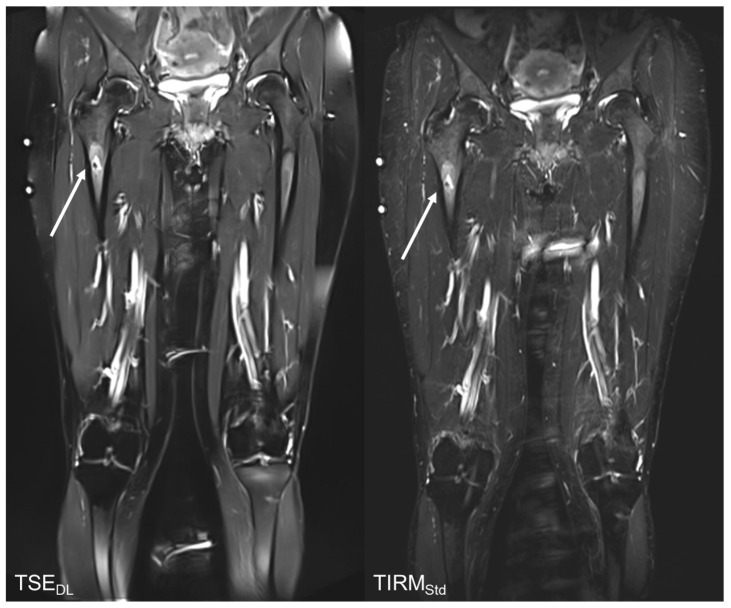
Follow up MRI examination of a 49-year-old with a T2w hyperintense lesion (arrows) of the right proximal femur, the finding would be compatible with enchondroma. The TSE_DL_ shows a better image quality, contrast, sharpness, and noise and thus, allows a better diagnostic confidence.
